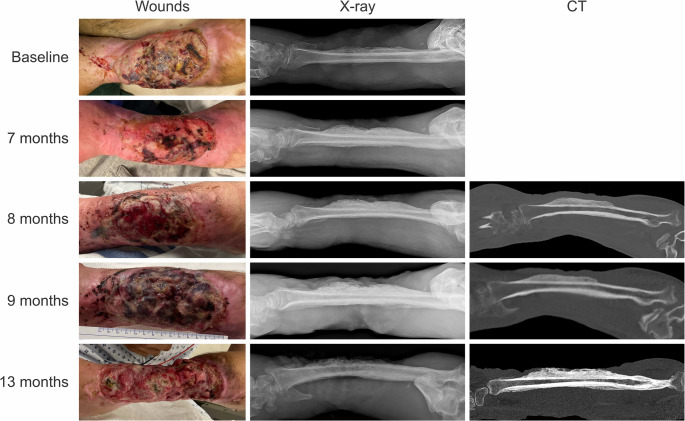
Longitudinal imaging in a 27-year-old man with an extensive soft-tissue wound of the right upper extremity associated with repeated xylazine injections. At baseline, radiographs demonstrated soft-tissue ulceration and periosteal reaction of the radial diaphysis, with segmental resection of the ulna involving the proximal and mid-diaphysis. Follow-up imaging demonstrated progressive radial periosteal reaction, with eventual exposure of the radial diaphysis through the overlying soft-tissue wound 13 months later, consistent with osteomyelitis
